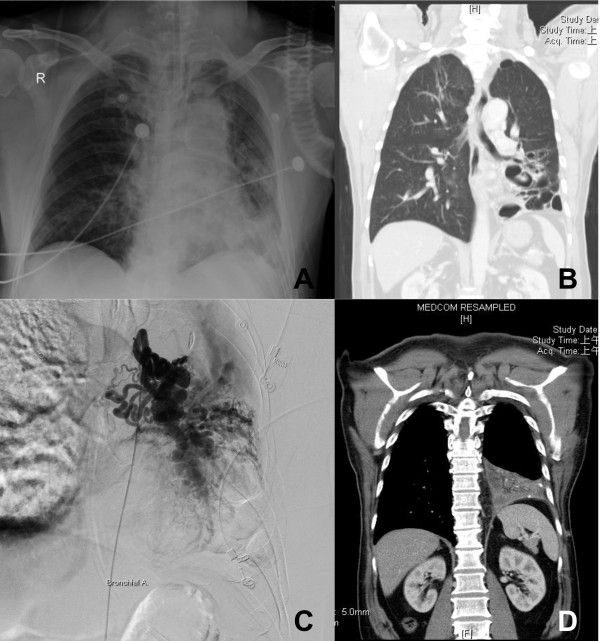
Some related images before and after the operation. A) Chest plain radiograph showed opacity in the left lower lobe with bronchial dilatation. B) Chest computed tomography (CT) revealed cystic dilatation in lingula and left lower lobe with numerous small vessels in the left hilar region. C) Angiography from the left bronchial artery revealed dilated and tortuous vessels. D) Chest CT 3 months postoperatively revealed consolidation of the left lower lobe and the absence of numerous prolifeating vessel.
